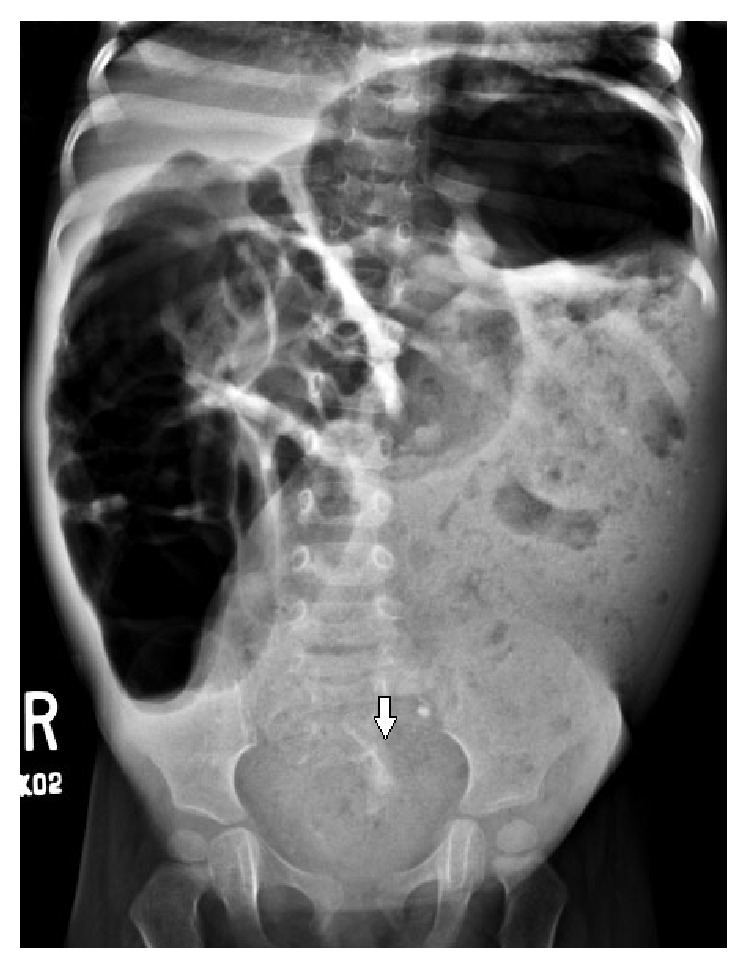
Abdominal radiograph for abdominal distention evaluation. It shows significantly dilated air and fecal filled bowel loops with fecaliths. There is a sickle-shaped sacral defect (arrow), not reported initially.
